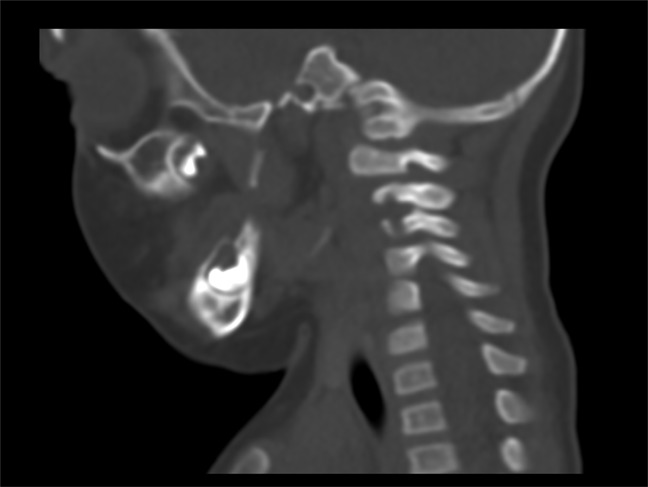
CT scan showing resolved tumoral calcinosis. CT scan of the patient at 11 months postoperatively showing resolution of the tumoral calcinosis.
